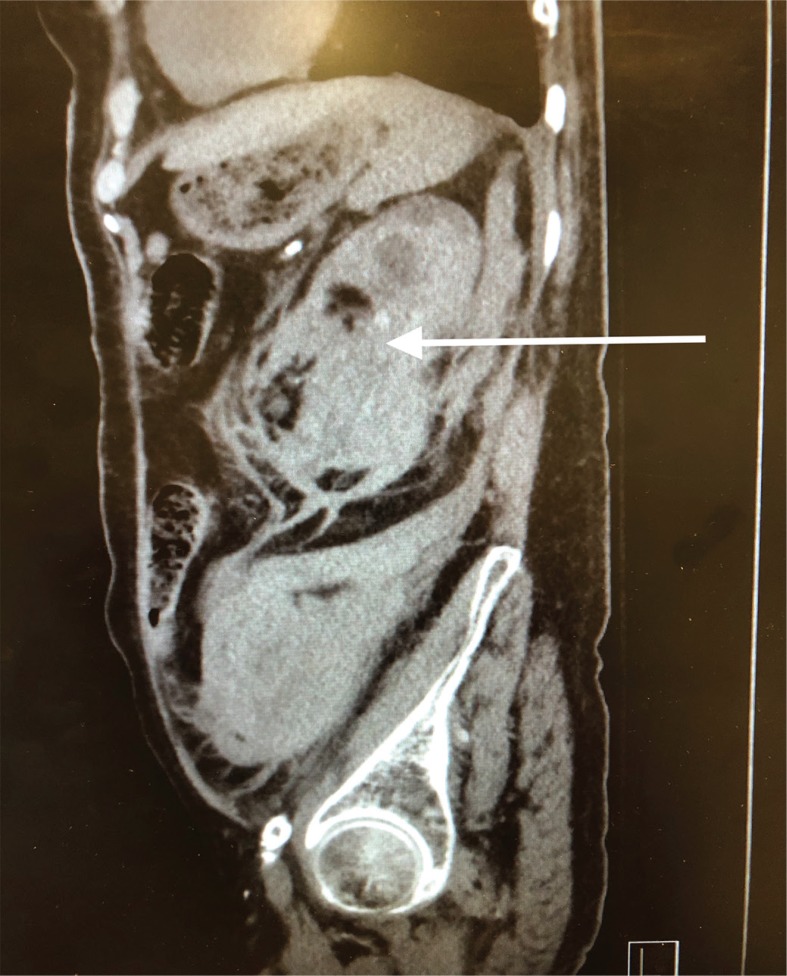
Sagital CT image of left retroperitoneal hemorrhage. The arrow shows the extensive left retroperitoneal hematoma with rupture of the left kidney.
